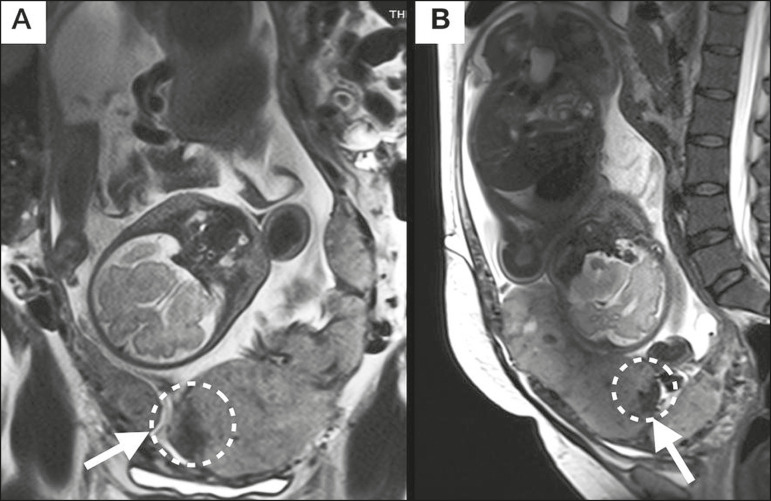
Coronal and sagittal T2- weighted MRI sequences (A and B, respectively) showing a placental recess (arrow) and dark bands (dotted circle).
